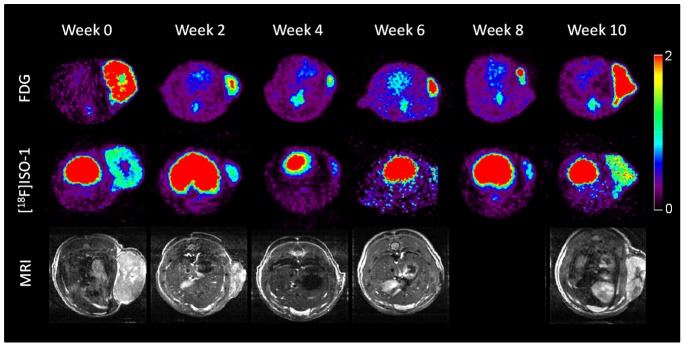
SUV 10-minute sum images at 60-minute post-injection of FDG (top row) and [^18^F]ISO-1 (middle row). Bottom row depicts the corresponding MR image at each time point (week 8 image is missing). Panel depicts treatment of tumor C1-1_L3 with bexarotene. Note that following the imaging session at week 8, treatment was withdrawn.
